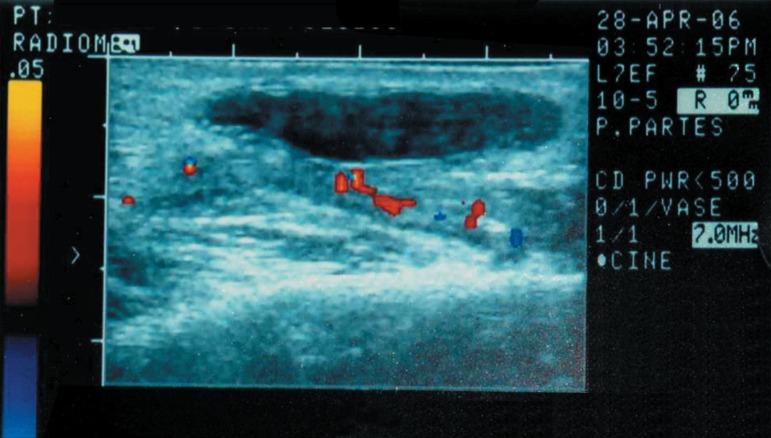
The longitudinal section in the ultrasonographic scan of the left parotid gland
